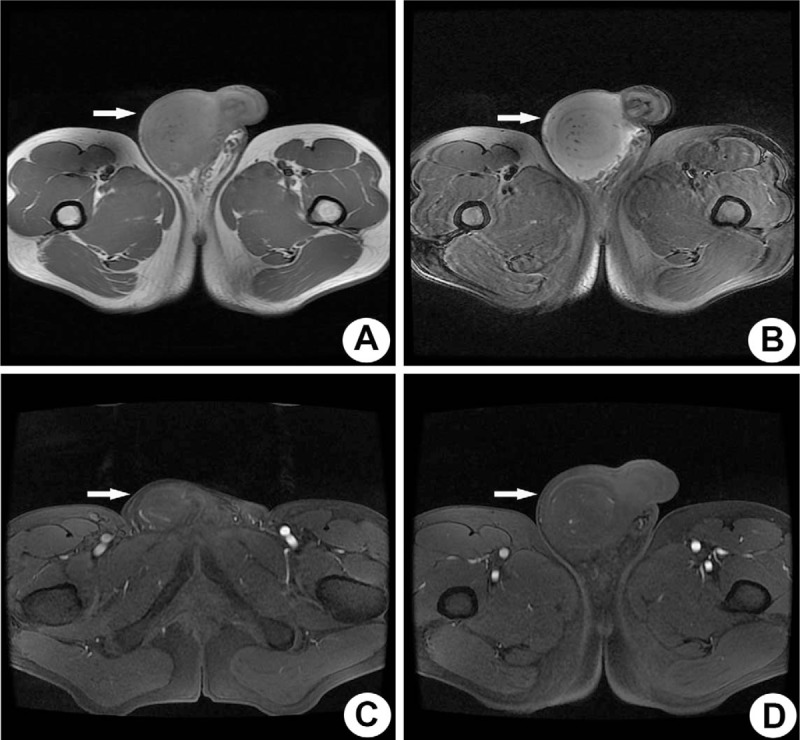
MRI images of primary testicular NK/T-cell lymphoma. (A) and (B) show a significantly enlarged right testicle due to a testicular mass, exhibiting an equal T1 and a slightly longer T2 signal, respectively. (C) and (D) show slightly inhomogeneous enhancement lines and dots in the mass. The lesion was highlighted with a white arrow.
